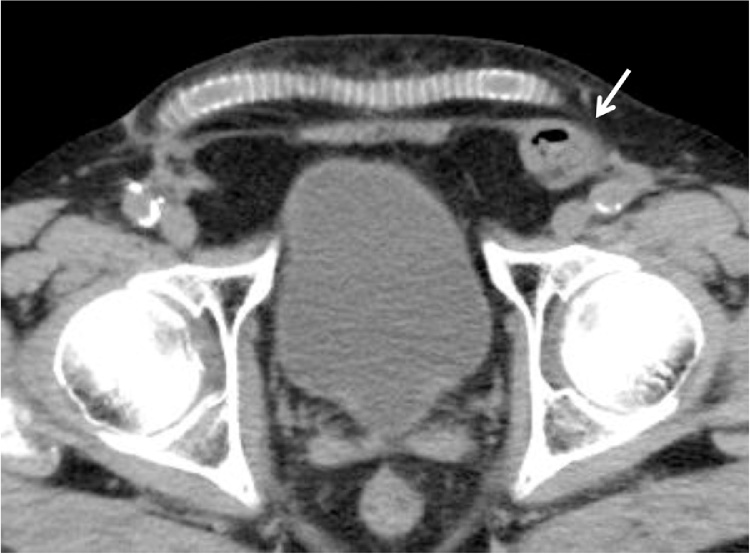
Case 1. Computed tomography revealed a left inguinal hernia (arrow) and femoro-femoral arterial bypass graft in the subcutaneous plane.
